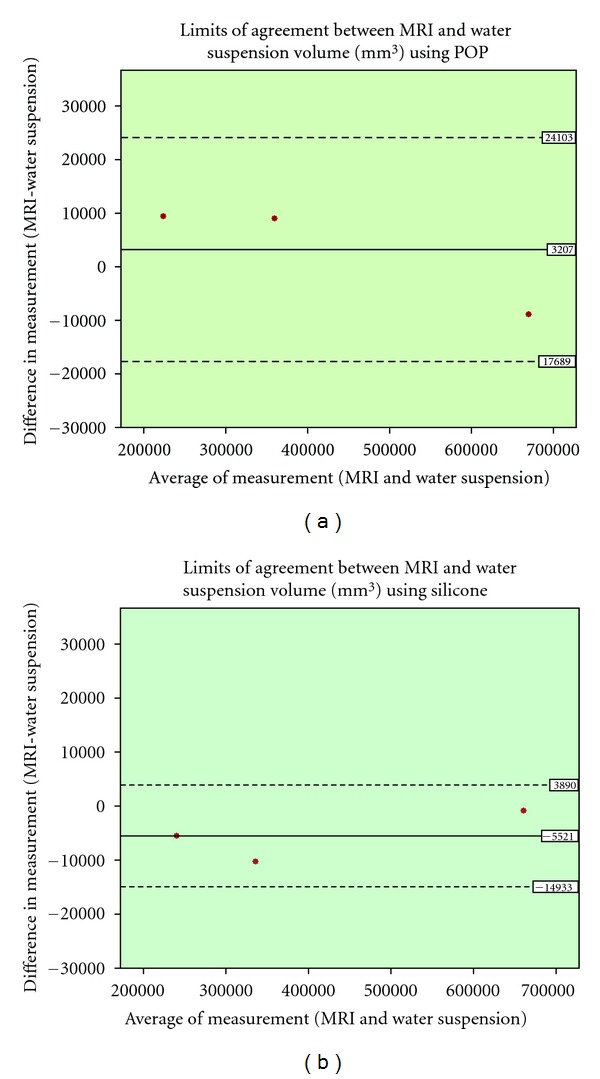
Bland and Altman plot for the volume measurement comparison of MRI and water suspension, (a): POP and (b): silicone.
